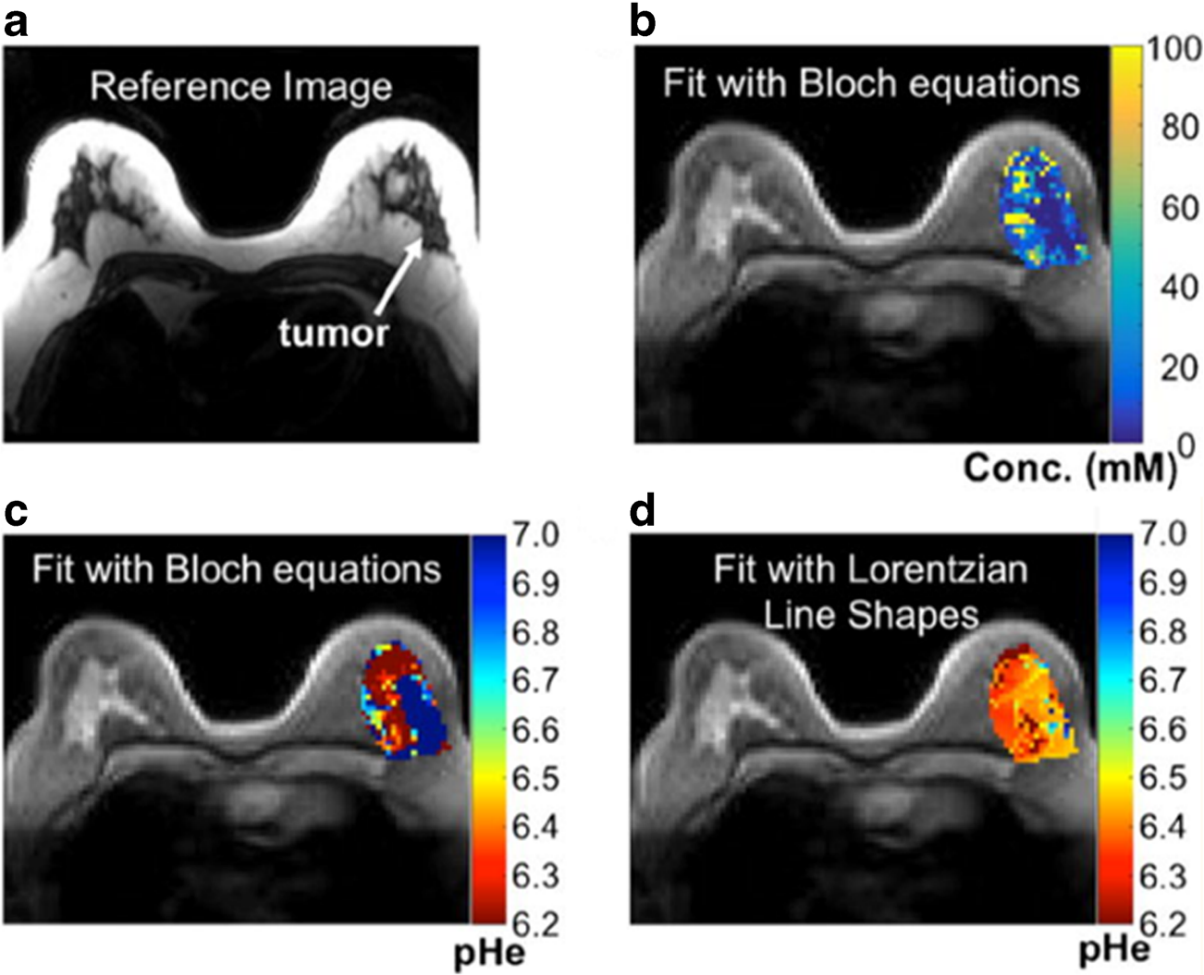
MRI-CEST imaging of tumor pH at clinical level. Representative patient with high-grade invasive ductal carcinoma and anatomical *T*_2_-weighted image (a) and parametric iopamidol concentration map (b) and tumor pHe map calculated by Bloch fitting (c) or by Lorentzian fitting (d) procedures overlaid on the anatomical image, showing, for the first time, tumor acidosis in patients. Adapted by permission from Springer Nature, Molecular Imaging and Biology 2017, 19, 617–625
